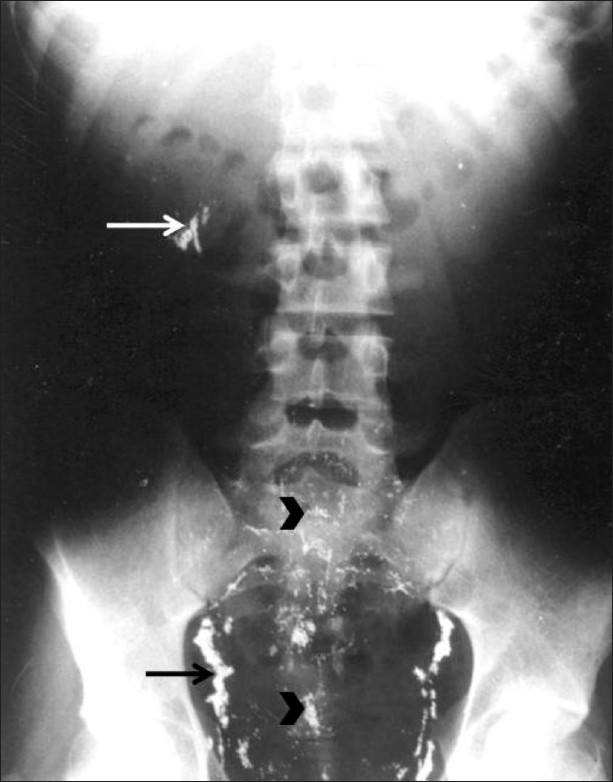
Follow-up radiograph of the abdomen shows tiny metallic densities in the region of the ureter and the pelvicalyceal system (arrows). Densities are also seen over the lower lumbar spine and the pelvis (arrowheads)
